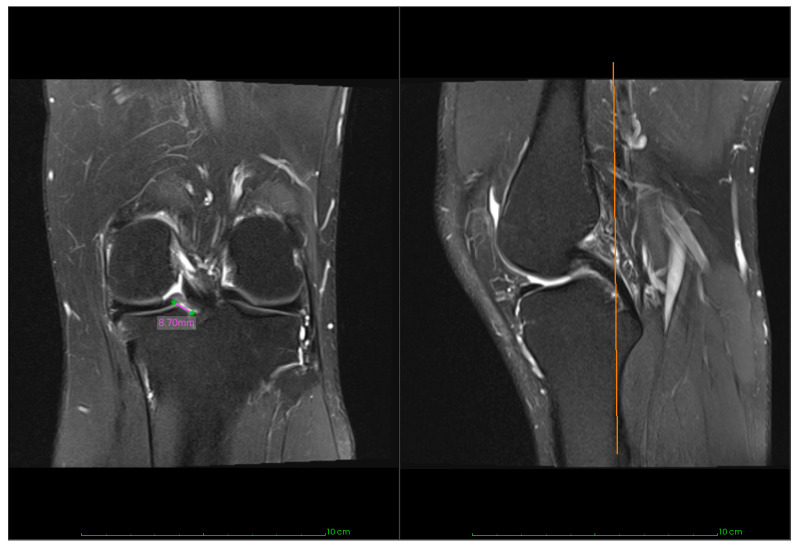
Measurement technique shown on proton density–weighted turbo spin-echo MRI with fat suppression, coronal (left) and sagittal (right) images, 3 mm slices: Straight-line distance from the anatomic medial posterior root attachment to the loose limb on the coronal slice (purple line); sagittal images are used to localize the root attachment relative to the posterior cruciate ligament tibial attachment (orange line).
